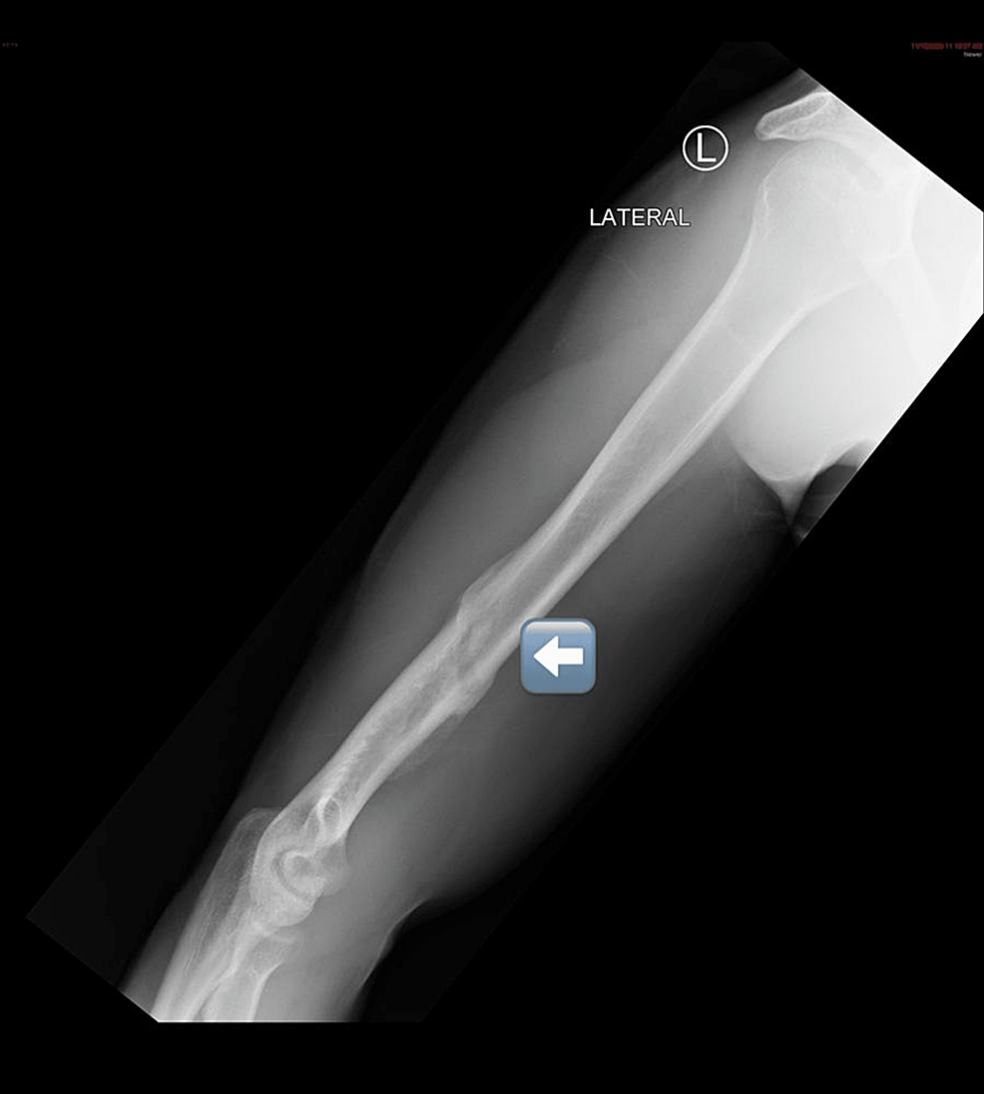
On the tenth-week visit, the X-ray showed well-healed and complete union
